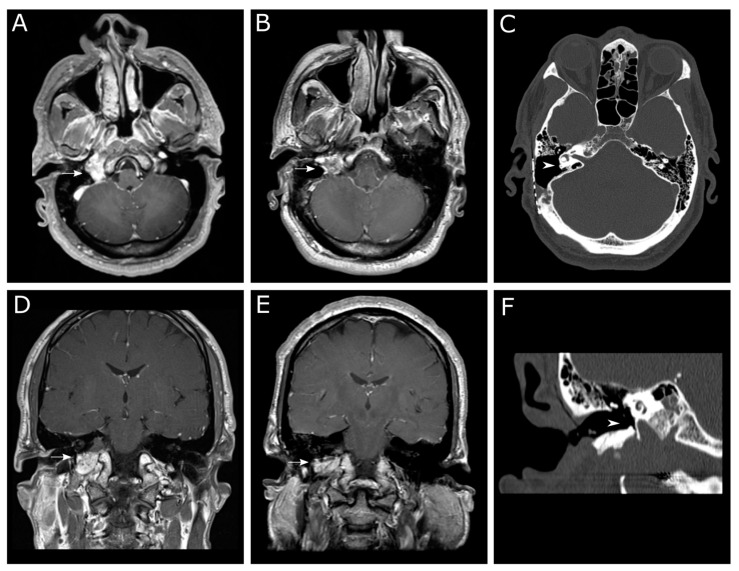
MRI and CT imaging of a patient with a Fisch class C jugular paraganglioma who underwent a modified infratemporal fossa approach for subtotal tumor resection for relief of pulsatile tinnitus and conductive hearing loss. Axial T1-weighted contrast-enhanced MRI presurgical (A) and postsurgical (B) imaging shows persistent tumor in the area of the jugular bulb. Axial CT bone window (C) postsurgical imaging shows no persistent tumor adjacent to the cochlea. Coronal T1-weighted contrast-enhanced MRI presurgical (D) and postsurgical (E) images show an interval decreased in tumor. Coronal CT bone window (F) postsurgical imaging shows no persistent tumor in the mesotympanum or hypotympanum. Arrows in (A,B,D,E) indicate tumor. Arrowheads in (C,F) show the absence of tumor in the middle ear.
